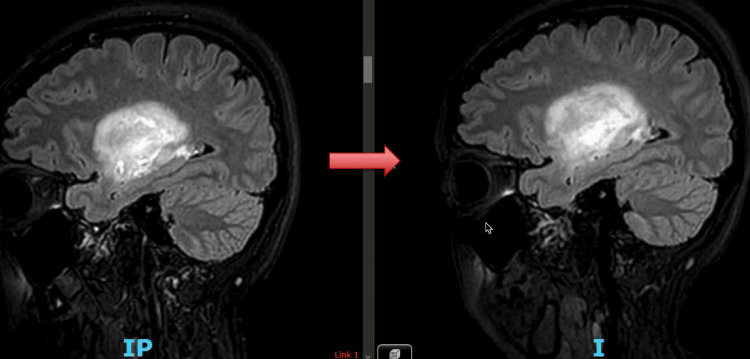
T2 FLAIR sagittal section of brain MRI showing extension of vasogenic edema on the right image after IVIG administration. FLAIR, fluid-attenuated inversion recovery; MRI, magnetic resonance imaging; IVIG, intravenous Immunoglobulin
